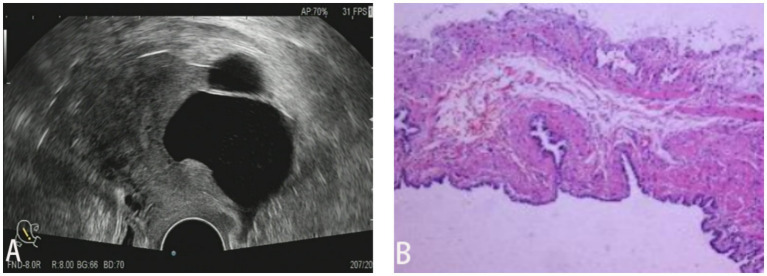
(A) Ultrasonography image and (B) postoperative pathological result of the second patient.
